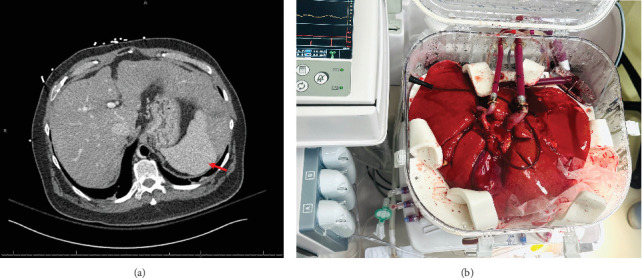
Preoperative liver assessment and intraoperative donor liver allograft preservation. (a) Preoperative axial contrast-enhanced computed tomography image demonstrating 1.3 × 2.9 cm hypoattenuating lesion of the left lobe of the liver (red arrow). (b) Normothermic machine perfusion of the donor liver on the Organ Care System (TransMedics).
